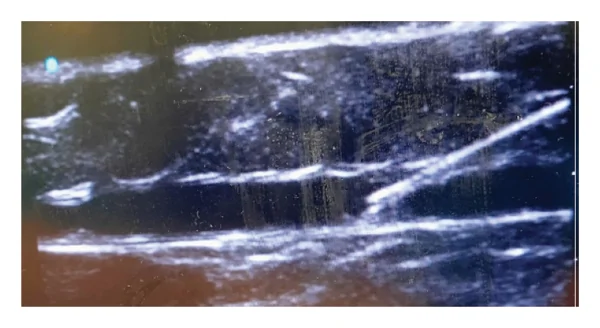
Ultrasound‐guided puncture of the right great saphenous vein.
